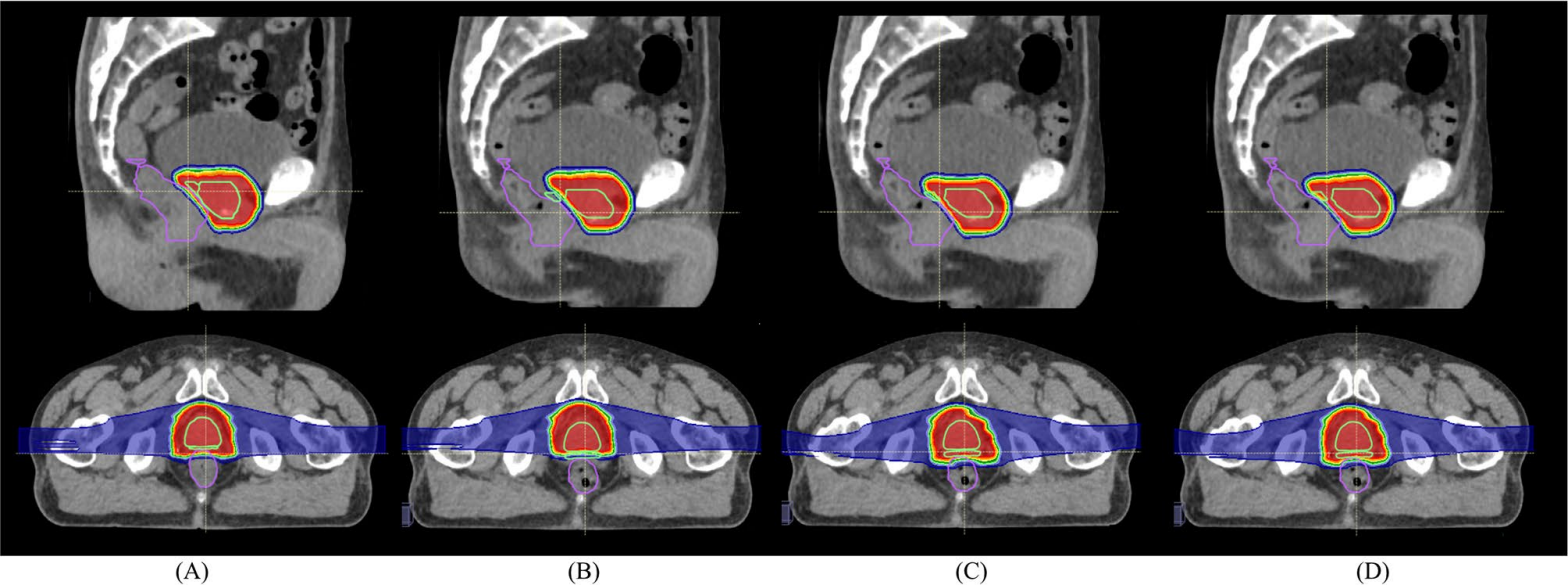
Dose distributions (case no.2) on (A) the planning CT and on the treatment CT (CT1) with (B) intensity-based image registration, (C) target-based image registration, and (D) WEPL-based image registration. Light green and purple lines outline the CTV and rectum, respectively.
